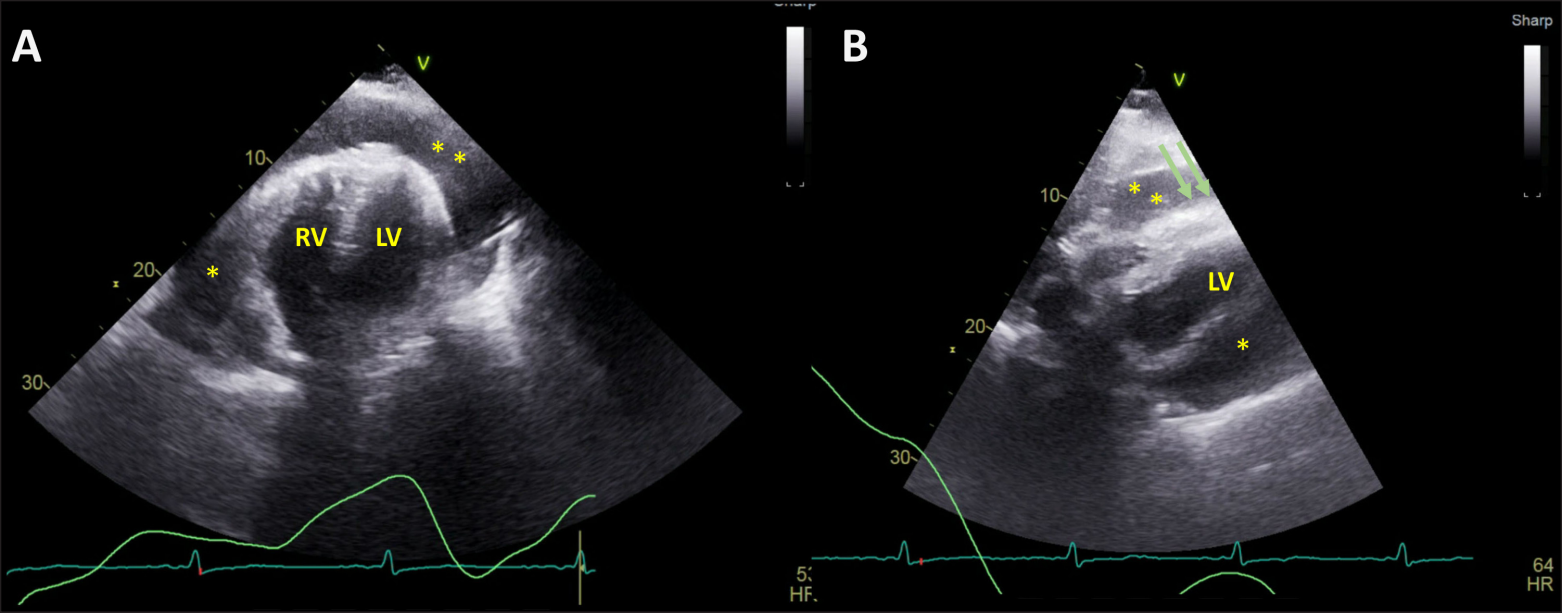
Transthoracic echocardiography, apical 4-chamber (A) and subcostal view (B) showing large circumferential pericardial effusion (asterisks) with right ventricular diastolic collapse (green arrows). LV: left ventricle; RV: right ventricle
